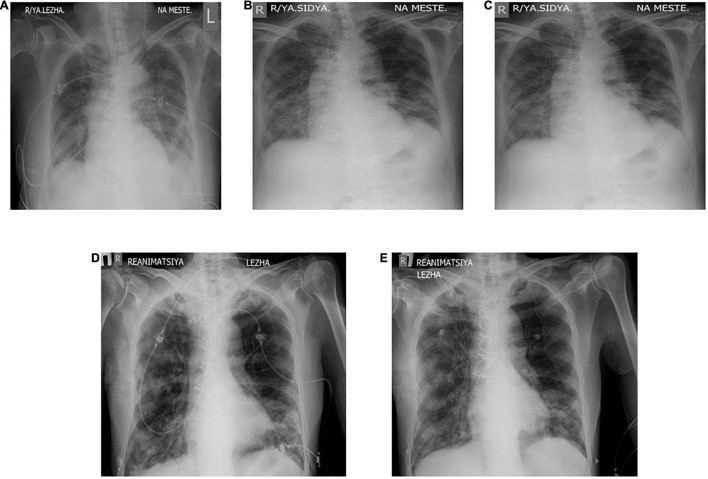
Dynamics of X-ray after the application of VALT: CXR on the 1st day (A), CXR after 18 sessions of VALT (B), CXR on the 16th day (BiPAP: FiO_2_ – 60%, f-18, I/E – 1/2, Pinsp – 25, PEEP – 14) (C), CXR on the 25th day (BiPAP: FiO_2_-40%, f-18, I/E – 1/2, Pinsp-17, PEEP-12) (D), CXR on the 32nd day (BiPAP: FiO_2_-40%, f-20, I/E – 1/2, Pins-20, PEEP 13) (E).
